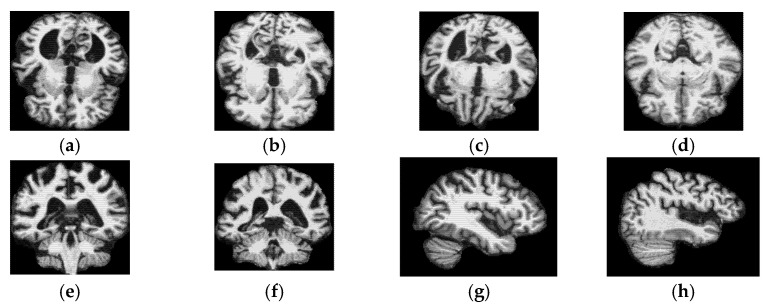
Samples of the selected 2D MRI slices. (a) AD—Segment 9; (b) CN—Segment 9; (c) AD—Segment 10; (d) CN—Segment 10; (e) AD—Segment 7; (f) MCI—Segment 7; (g) CN—Segment 4; (h) MCI—Segment 4.
